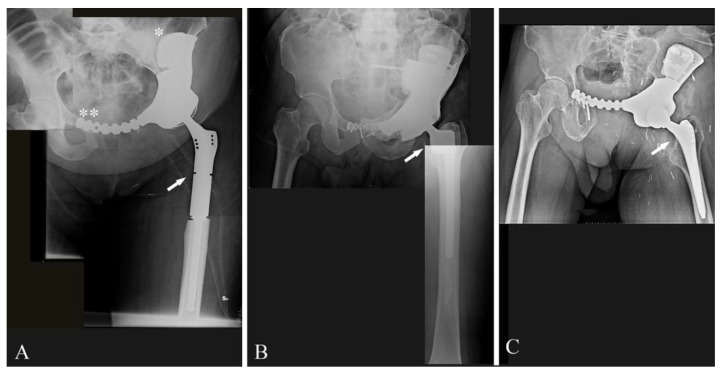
(A) Post-operative X-ray showing reconstruction after G1 chondrosarcoma of the left hemipelvis with a 3DPTCMP fixed to the residual iliac bone with a “wafer” system (white asterisk) and then to the residual symphysis with a plate (double white asterisk); proximal femur was reconstructed with a modular prosthesis and a constrained cup (white arrow) (case 1); (B) post-operative X-ray showing reconstruction after resection of a left hemipelvis G2 chondrosarcoma; reconstruction was performed with a short modular femoral prosthesis (white arrow) and a constrained cup cemented in a 3DPTCMP (case 5); (C) post-operative X-ray showing reconstruction after resection of a left hemipelvis G3-Chondrosarcoma; reconstruction was performed with a standard femoral stem prosthesis (white arrow) and a constrained cup cemented in a 3DPTCMP (case 12).
